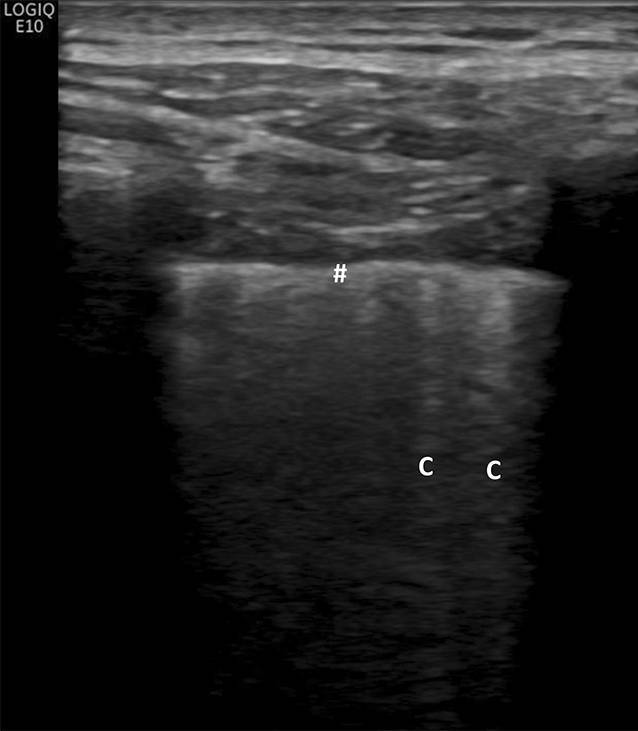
Die diagnostische Wertigkeit des transthorakalen Ultraschalls für SSc-ILD wurde in einer Reihe von wissenschaftlichen Arbeiten untersucht (Tab. 1 und 2) und gegen den Goldstandard CT verglichen. Hierbei wurden die CT-Untersuchungen mit dem Warrick-Score ausgewertet und dem transthorakalen LUS gegenübergestellt. Der Warrick-Score ist eine semiquantitative Scoring-Methode, um das Ausmaß und die Schwere der SSc-ILD in der Computertomographie zu beschreiben [41].
